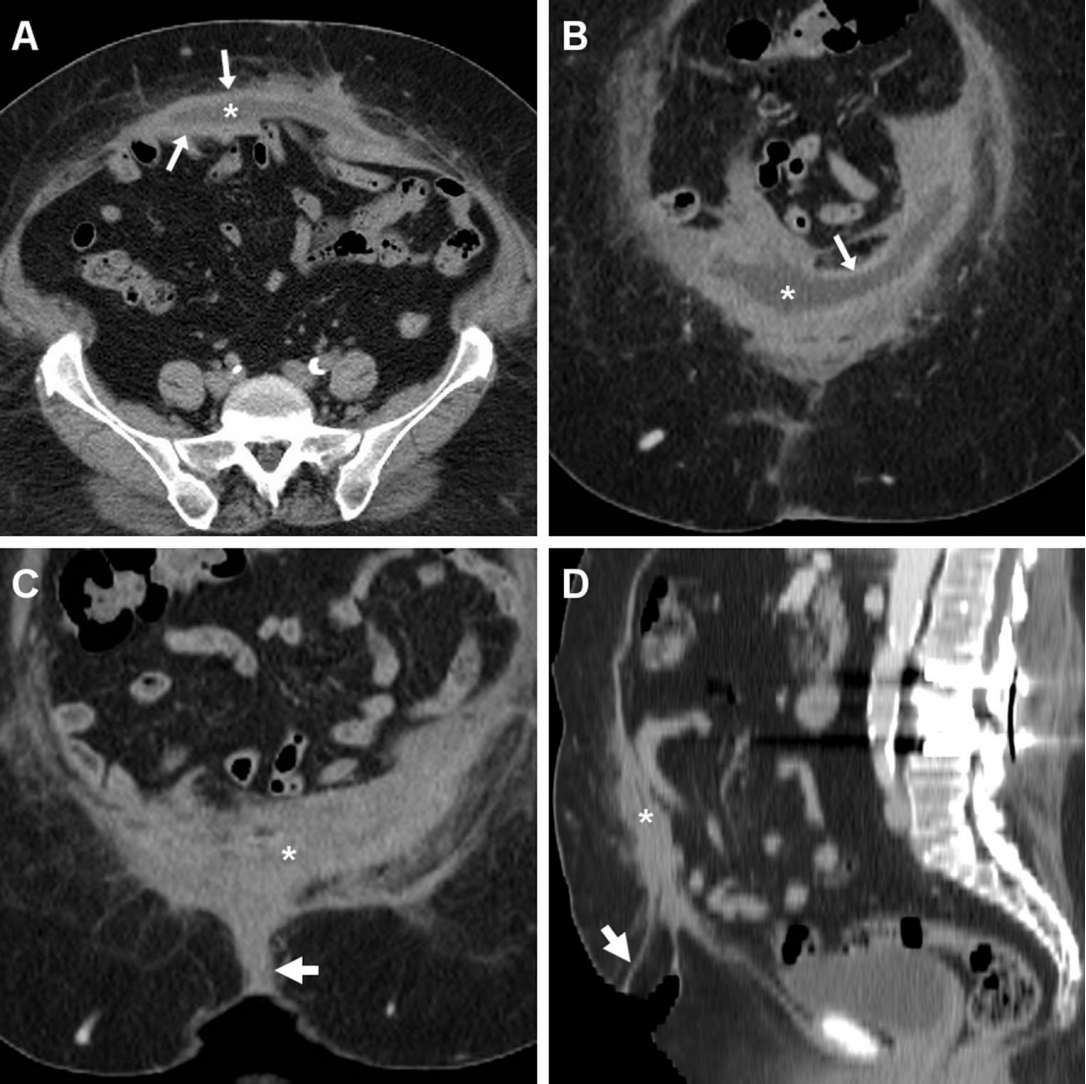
In a 75-year-old female, after recent open repair of post- hysterectomy incisional hernia, axial (a), coronal (b), and oblique-coronal (c) multidetector CT images depicted a crescent-shaped abscess of the anterior abdominal wall (*) with enhancing periphery, connected to the cutaneous opening by a fistula (thick arrows). Surgical reintervention with PM removal and replacement was required
